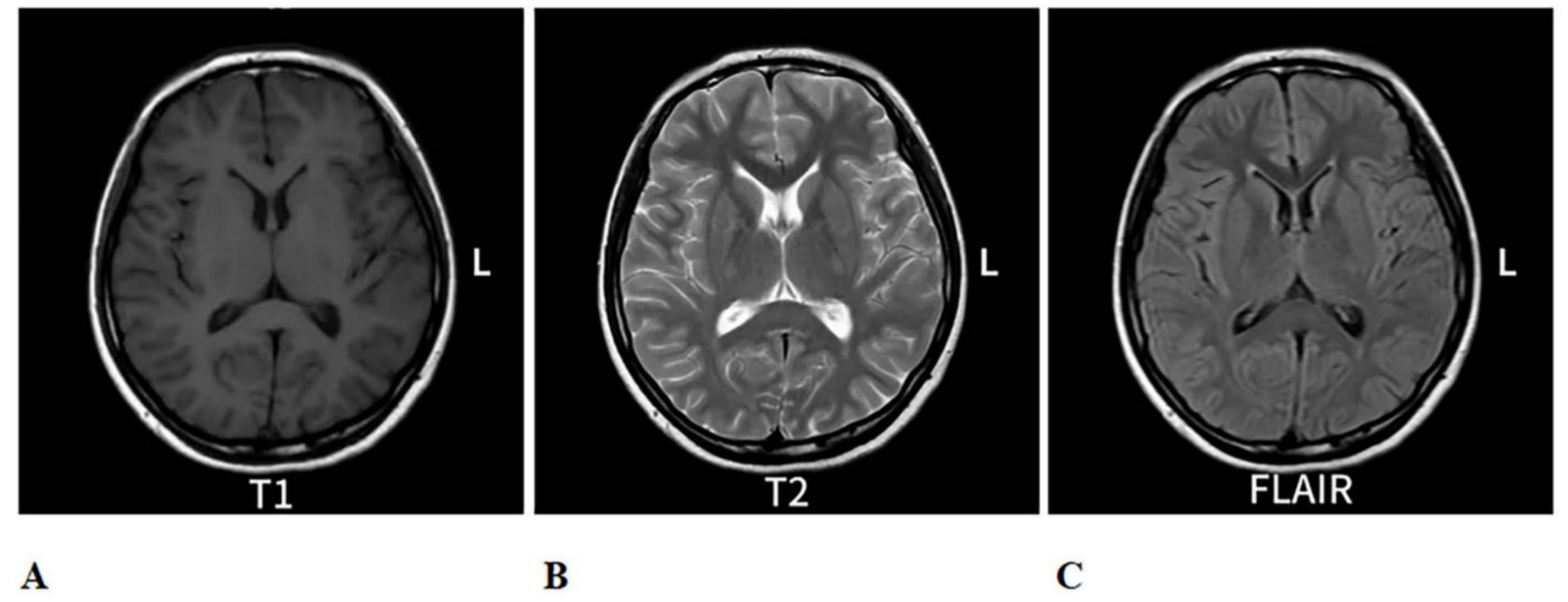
Re-evaluation of cranial MRI on 6 August 2024, compared to the MRI from 25 July 2024: The abnormal signal in the splenium of the corpus callosum. (A) is a T1-eighted image with low contrast. (B) is a T2-weighted image showing higher ontrast, highlighting fluid. (C) is a FLAIR image with intermediate contrast, uppressing fluid signals. Each scan shows axial brain views with symmetrical features.
